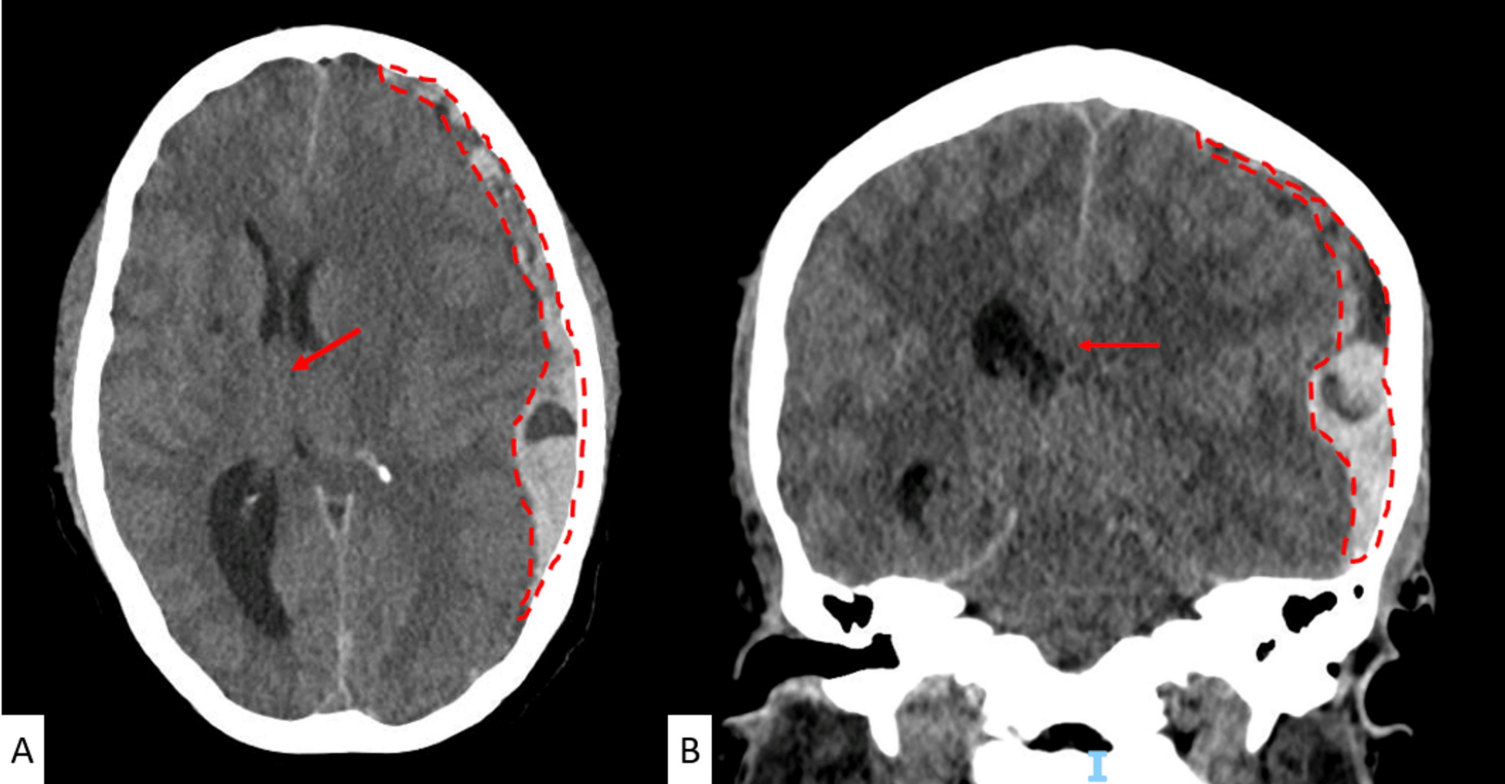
CT scan of the cranium (axial view (A) and coronal view (B)) with evidence of a subdural hematoma in the left hemisphere (red dashed line) with significant midline shift (red arrow) and uncal herniation.
